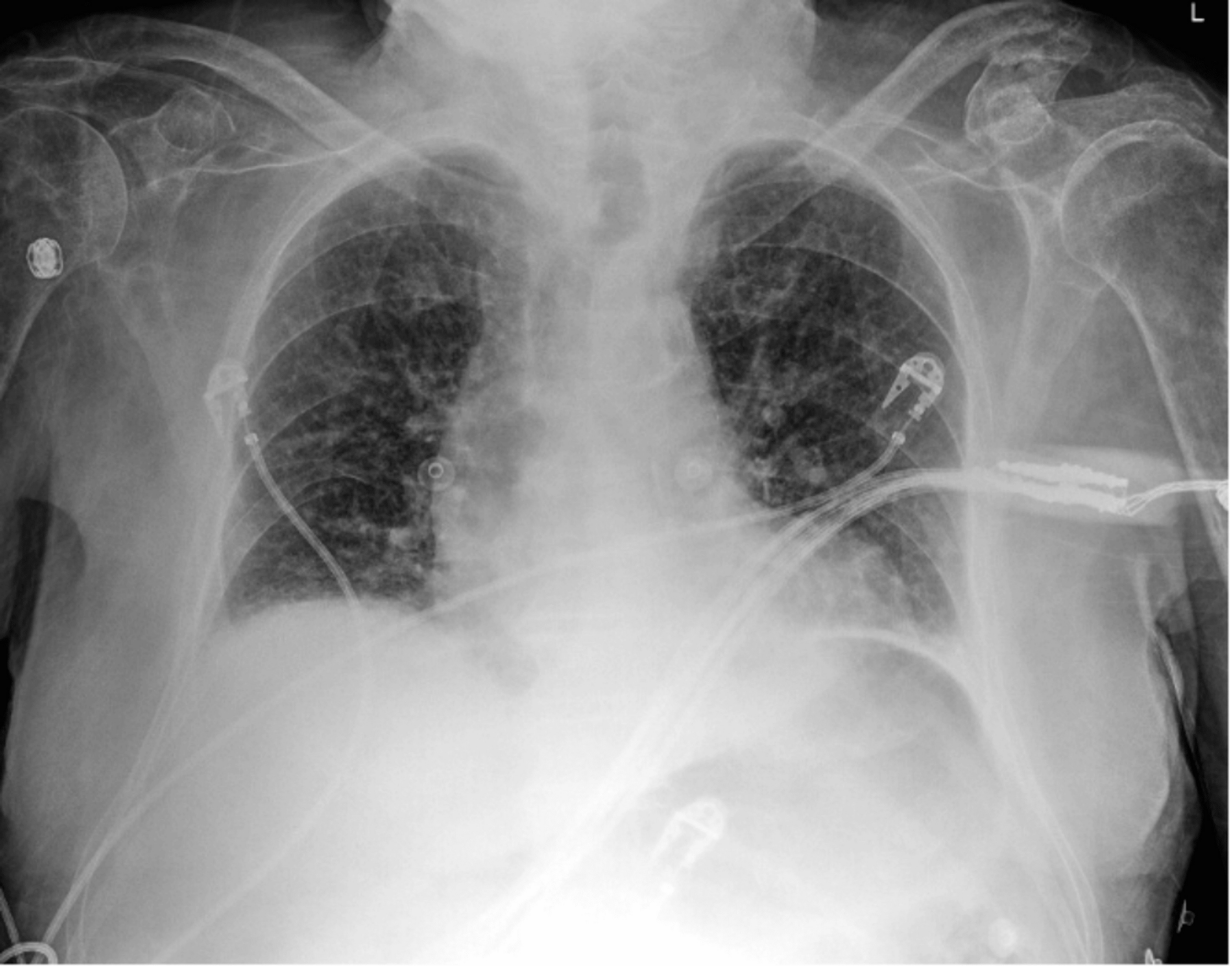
Chest X-ray showing mild edema, pulmonary venous congestion, and left basilar atelectasis.
